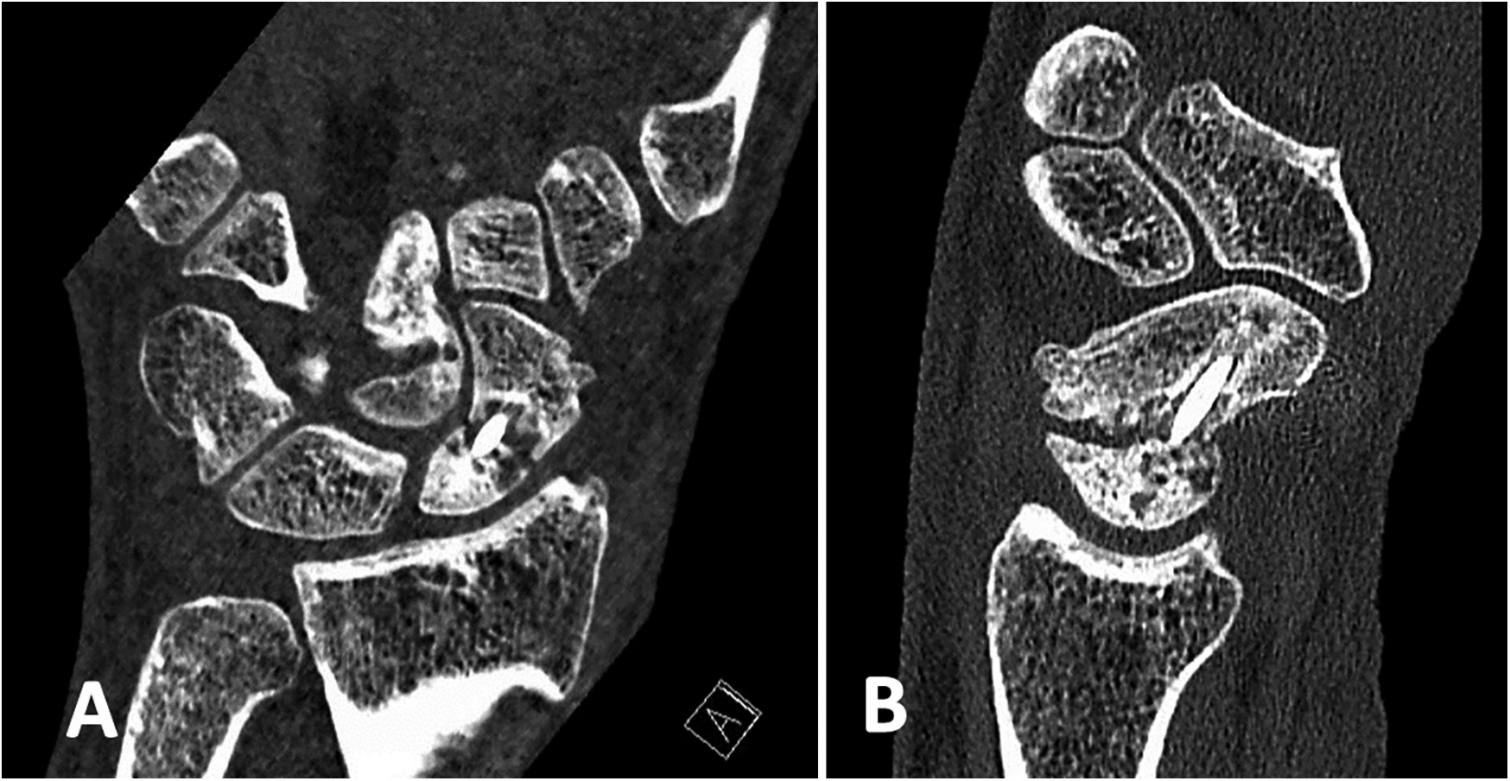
Preoperative CT scans of the patients 1.5 years after a failed osteosynthesis with compression screw showing a site of nonunion: (A) in coronal and (B) in sagittal projections. Scaphoid reconstruction has been performed (See Figure 2).
